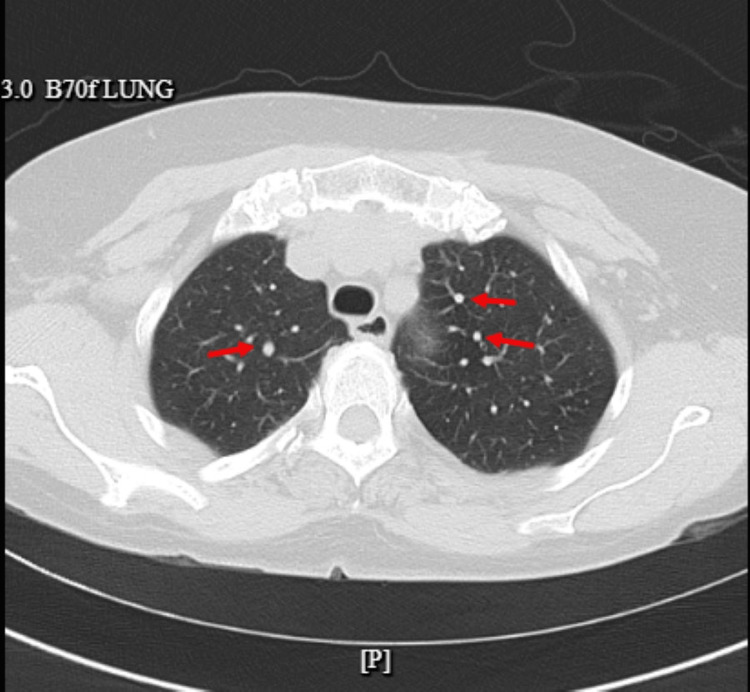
CT of the chest showing multiple pulmonary nodules as pointed out by the red arrows
